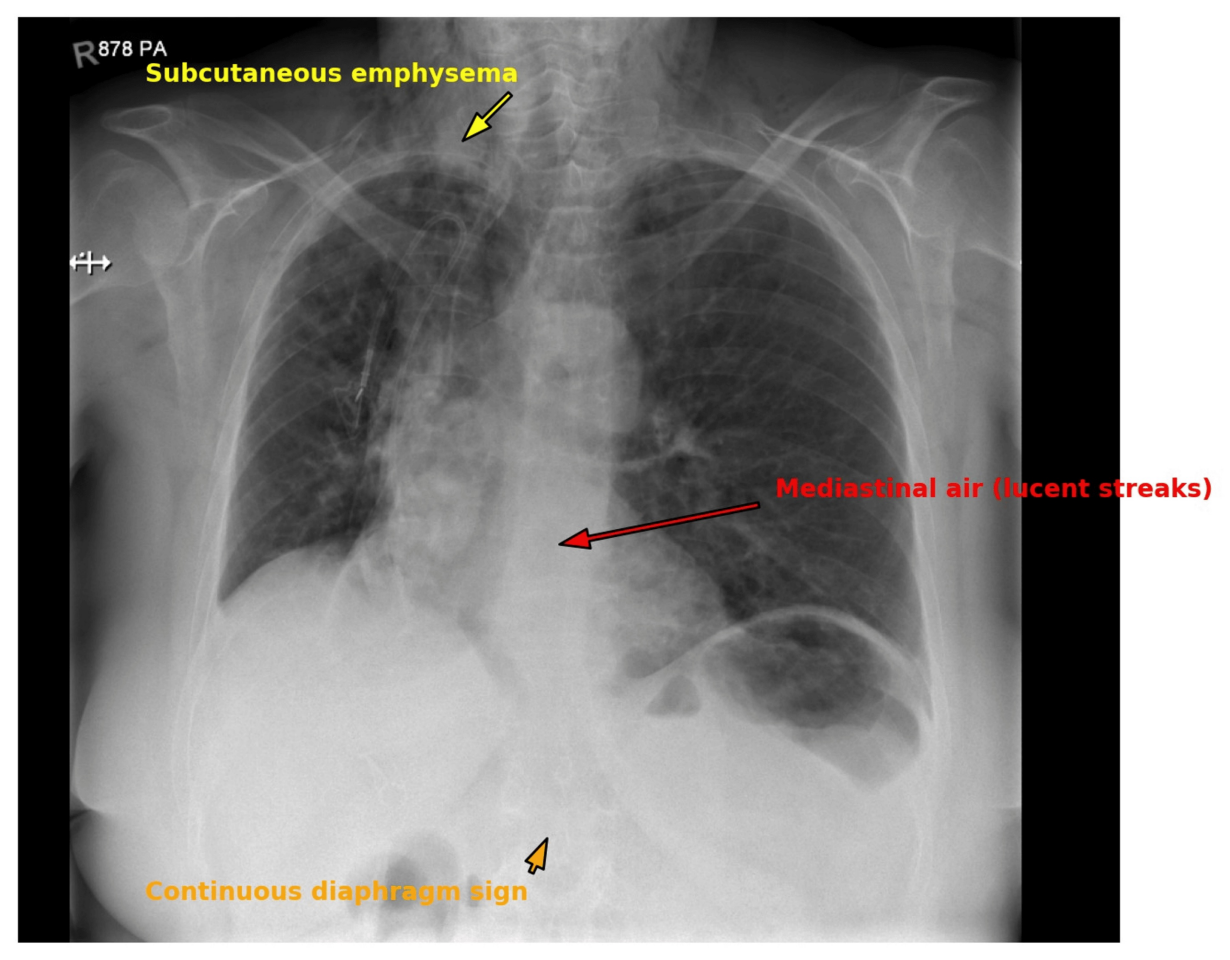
Chest X-ray. Posteroanterior chest X-ray showing pneumomediastinum. Subcutaneous emphysema is seen in the upper chest and neck (yellow arrow). Mediastinal air outlines mediastinal structures (red arrow), with a continuous diaphragm sign (orange arrow) beneath the heart. No pneumothorax is observed.
